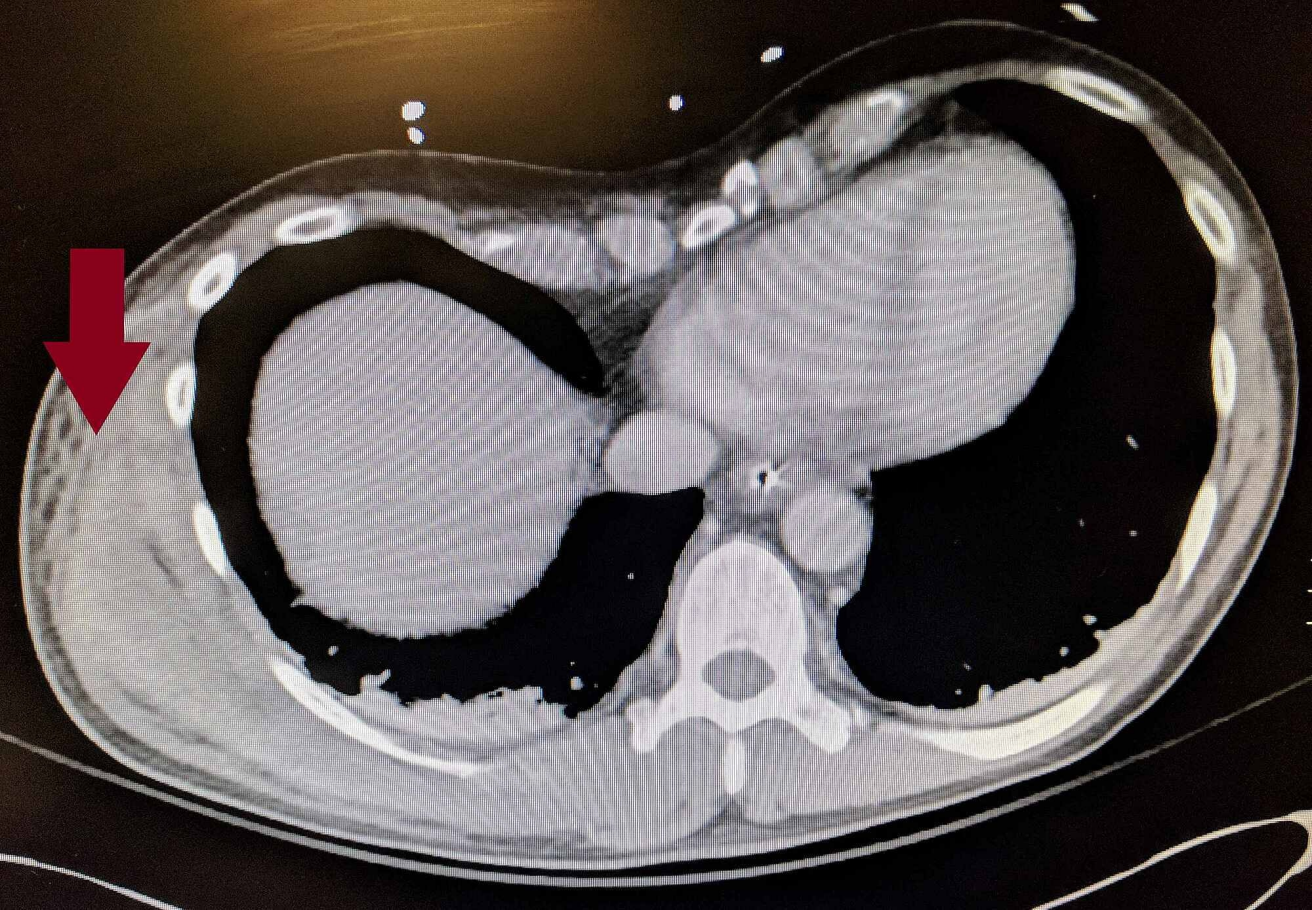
CT chest, abdomen, pelvis revealing fluid in the fascial planes in the right lateral chest wall.
